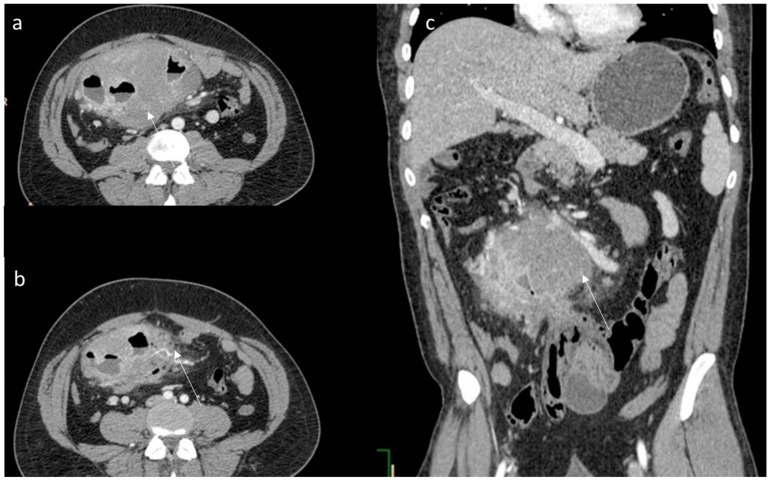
(a,b) CT assessment (arrow) of aggressive fibromatosis; the arrow shows lesion in axial; (c) MPR coronal plane of portal phase of contrast study. The lesion enhancement is mild to moderate with involvement of intestinal loops.
